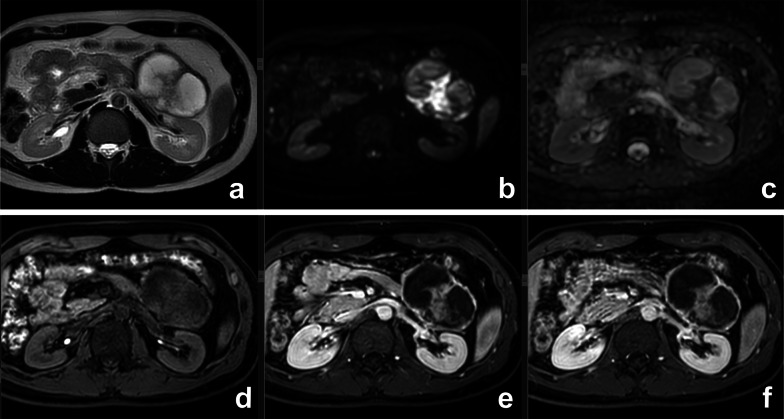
Solid pseudopapillary neoplasm. On T2w (a), a large cystic lesion with solid components is appreciable in the tail of the pancreas. The solid component shows high signal on DWI b 800 (b) and restricted diffusion at ADC map (c) and contrast enhancement on T1w after paramagnetic contrast media administration (d–f)
